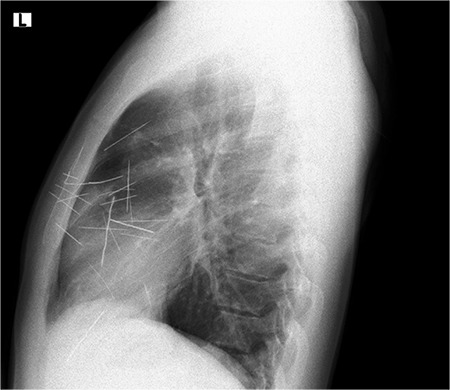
Thin, linear, metallic opacities superpose to sternum, middle and anterior mediastinum and diaframe in chest lateral X-ray.
